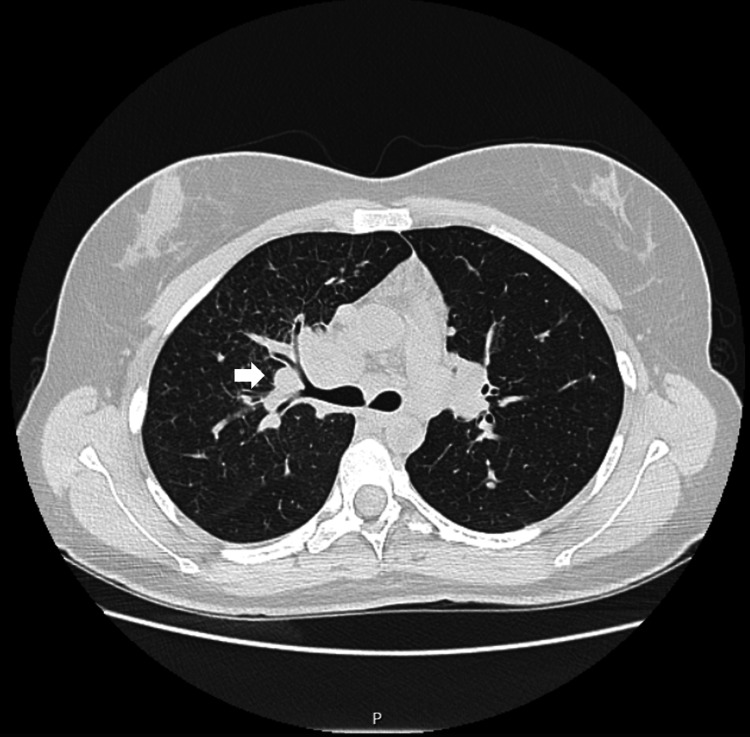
First chest computed tomography scan showing the hilar lymphadenopathy clusters marked with an arrow (May 28, 2024).
